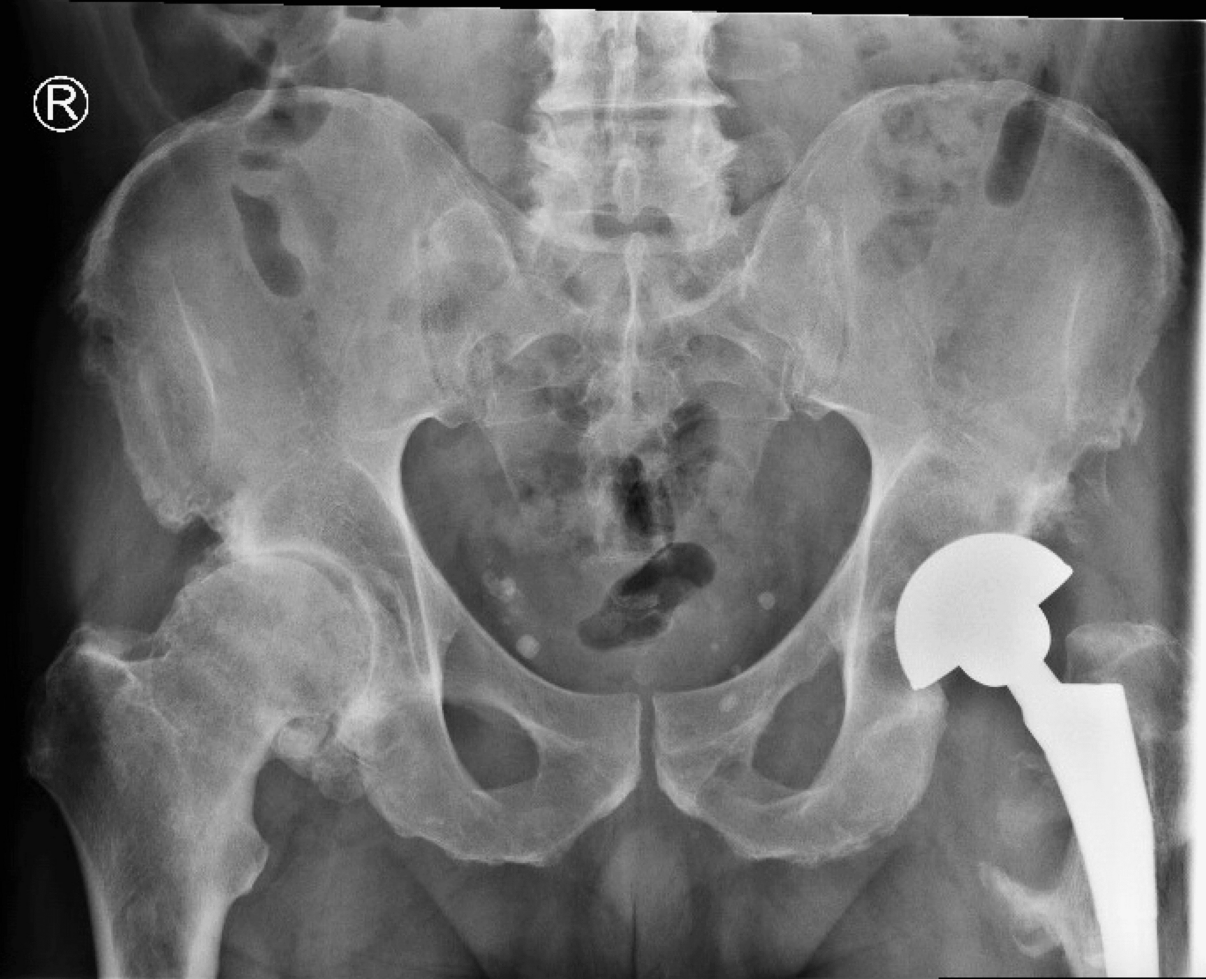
Anterior-posterior pelvis radiograph of revised left total hip arthroplasty with polyethylene liner and ceramic head.
